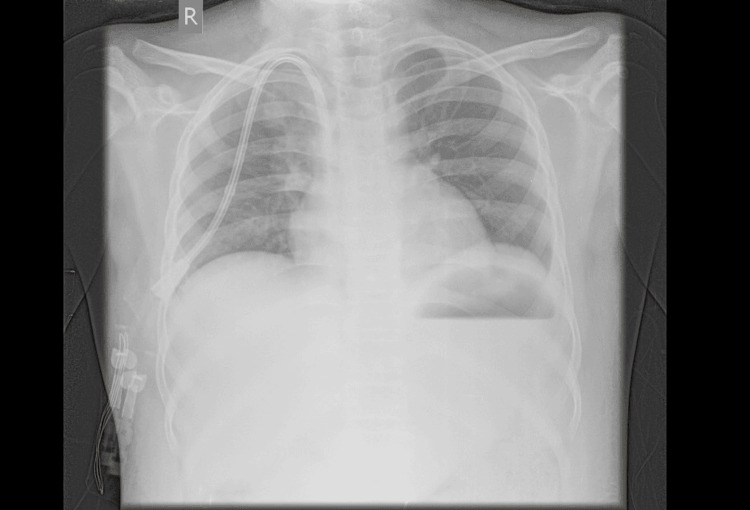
PA view of chest radiograph PA: Posteroanterior
